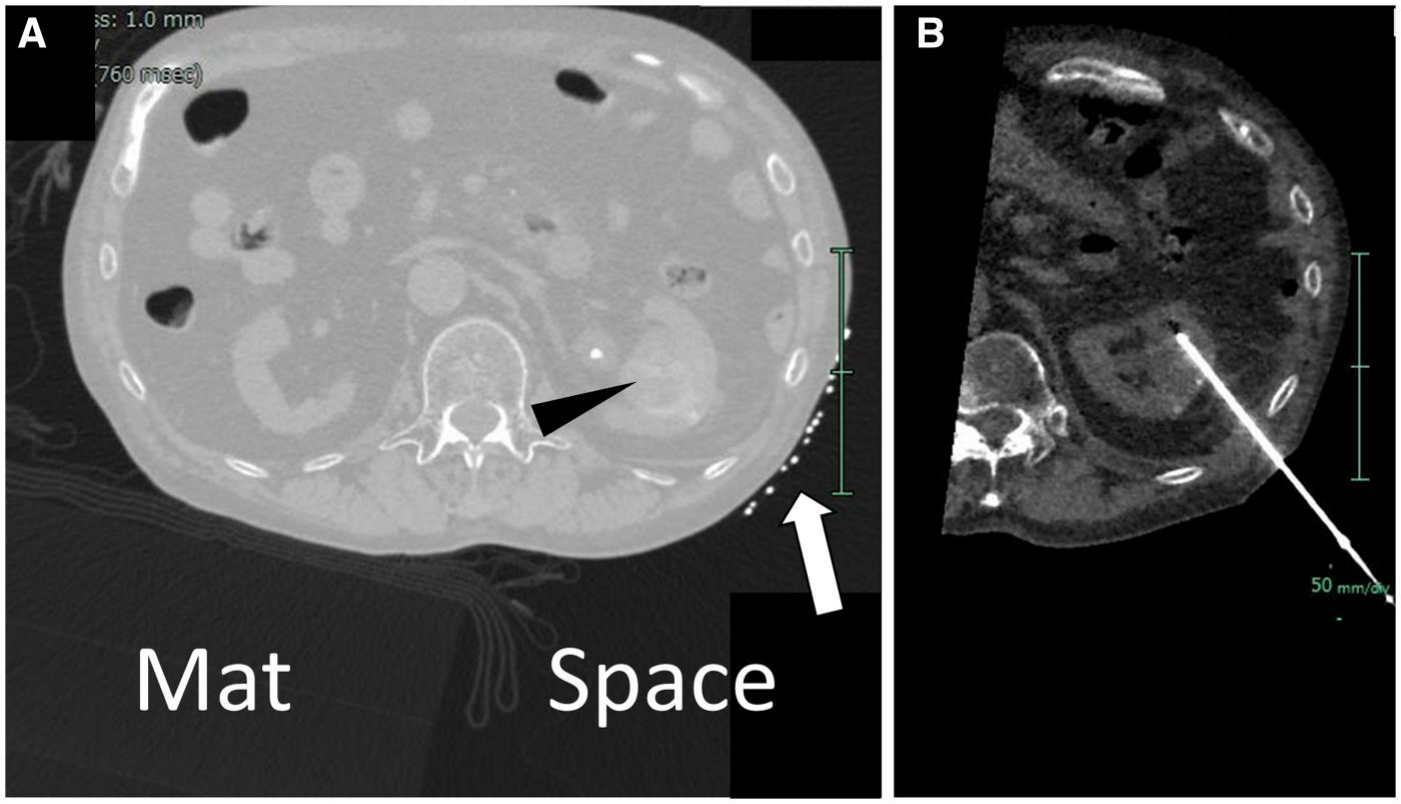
A 69-year-old man undergoing RFA for a left renal tumour. (A) CT image of puncture planning (pulmonary window setting). The polyorefin mat is thinly visible in the pulmonary window setting, revealing the presence of a procedural space. The patient is supine, and a marker (arrow) is placed on the left lateral back. Arrowhead: left renal tumour. (B) CT image during CT fluoroscopic guidance. The RF needle is inserted into the left renal tumour through the procedural space.
